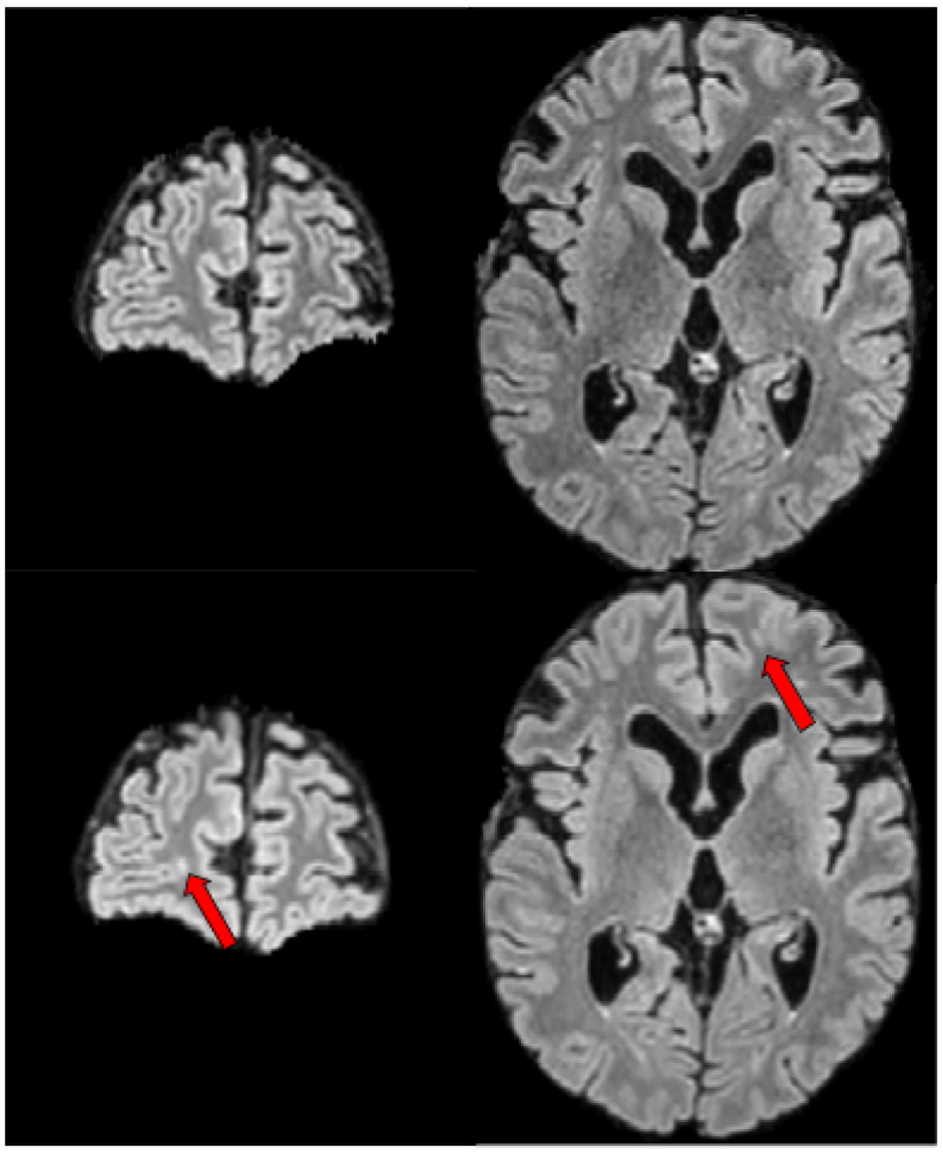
Example of a lesion detected by none of the experts in the first phase of the experiment, detected by the segmentation module and accepted by all experts in the second phase of the experiment. First row shows the baseline FLAIR scan (from left-to-right: coronal and axial view), second row shows the FLAIR scan 1 year later (from left-to-right: coronal and axial view). Red arrows designate the lesion of interest.
